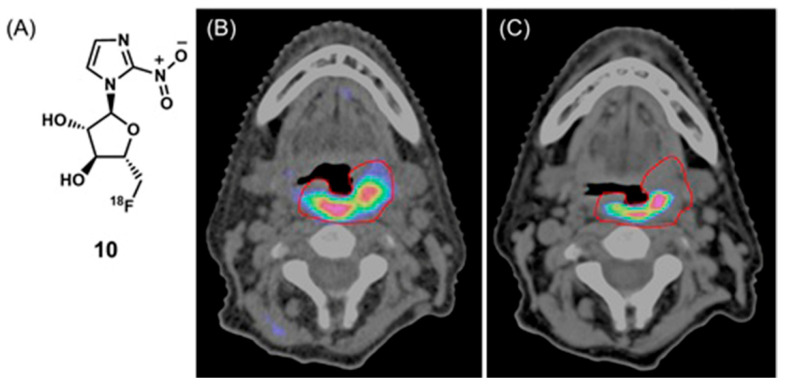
(A) Chemical structure of 10. (B) PET–X-ray CT scan of a patient with oropharyngeal cancer before radiation therapy. The red solid line indicates the gross tumor volume. (C) Hypoxic volume after 12 Gy radiation therapy. After radiation therapy, the hypoxic volume decreased from 30.9 to 13.7 cm^3^. Adapted from *Radiotherapy and Oncology*, *105*, 1, Mortensen, L. S.; Johansen, J.; Kallehauge, J.; Primdahl, H.; Busk, M.; Lassen, P.; Alsner, J.; Sørensen, B. S.; Toustrup, K.; Jakobsen, S.; Petersen, J.; Petersen, H.; Theil, J.; Nordsmark, M.; Overgaaerd, J. FAZA PET/CT hypoxia imaging in patients with squamous cell carcinoma of the head and neck treated with radiotherapy: results from the DAHANCA 24 trial. 14–20, Copyright 2012, with permission from Elsevier [275].
